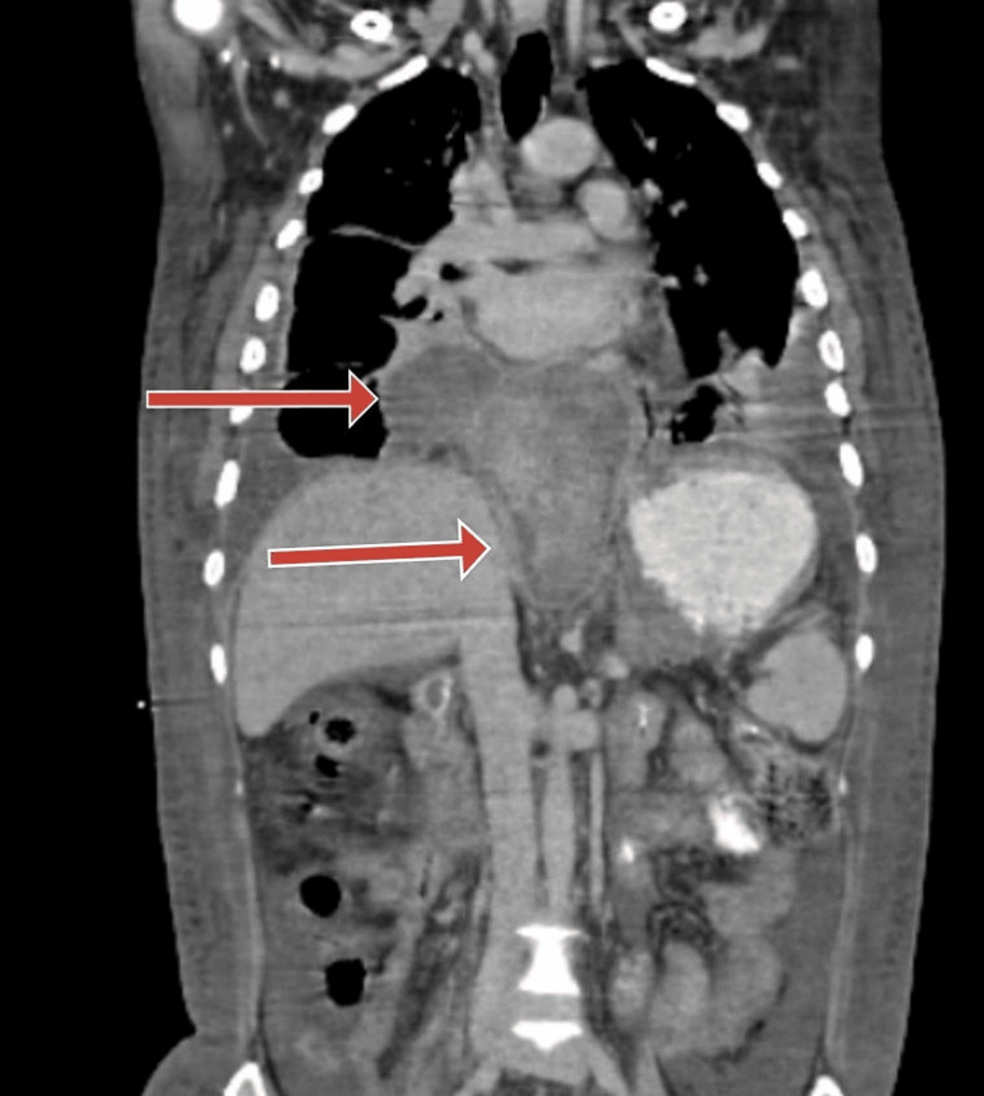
Contrast-enhanced computed tomography imaging of abdomen revealed the pancreatic pseudocyst with mediastinal extension (red arrows).
